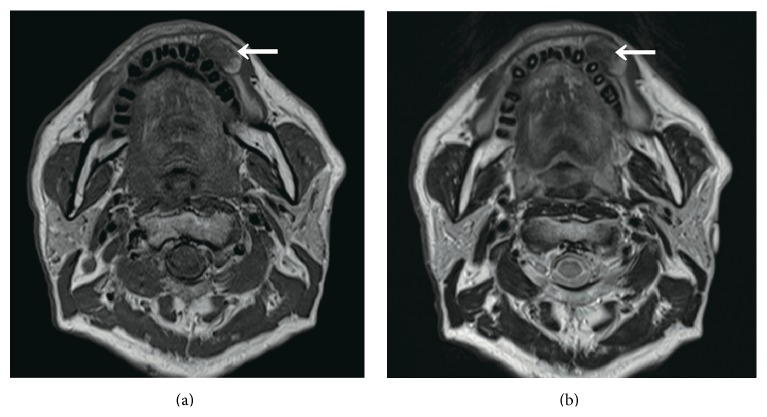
MRI findings of the lesion. MRI showed a well-defined mass that exhibited low signal intensity on both (a) T_1_- and (b) T_2_-weighted images.
